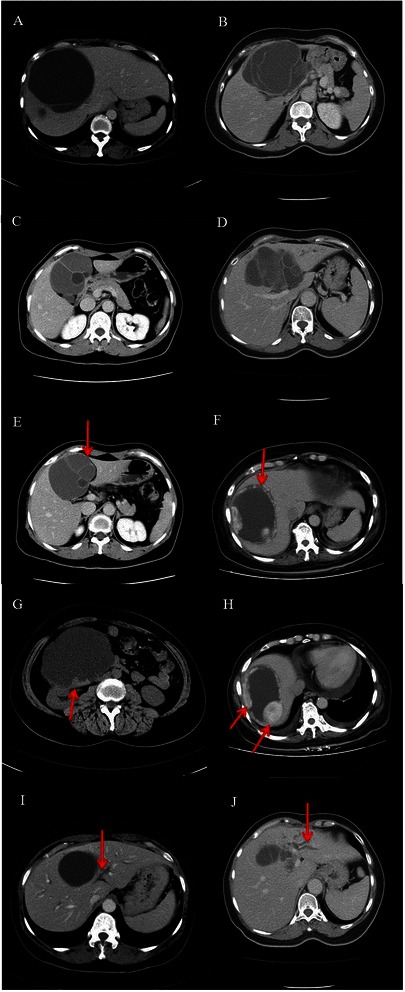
Contrast-enhanced computed tomography (CE-CT) of patients with intrahepatic biliary cystadenomas. a and b, internal septation: cystic lesion was divided by one or more septa. c and d, loculation: the presence of small spaces or cavities within the main cystic lesion. e and f, cystic wall enhancement: the enhancement of cystic wall after contrast administration. g and h, wall thickening and/or mural nodules: the presence of cystic wall nodules or focally thickened cystic wall. I and J, biliary dilatation: the presence of dilated intrahepatic ducts due to intrahepatic cystic lesion
